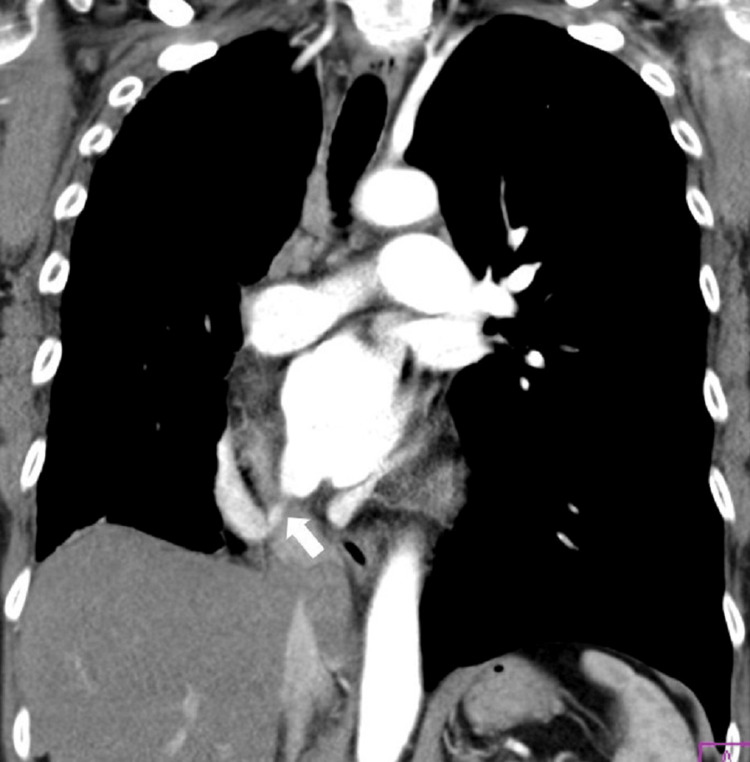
Initial CT image: the re-implanted solitary right pulmonary vein with a critical stenosis at the left atrial confluence (arrow).
